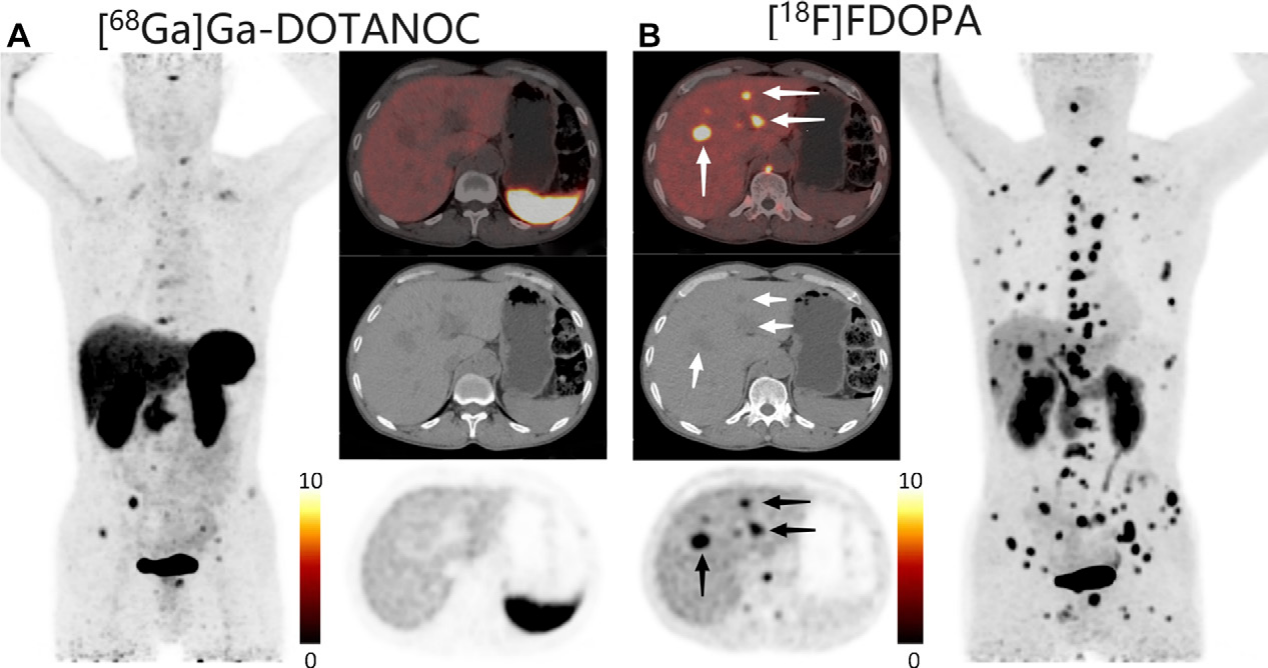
PET/CT scans in a 29-year-old female patient who underwent surgery for
